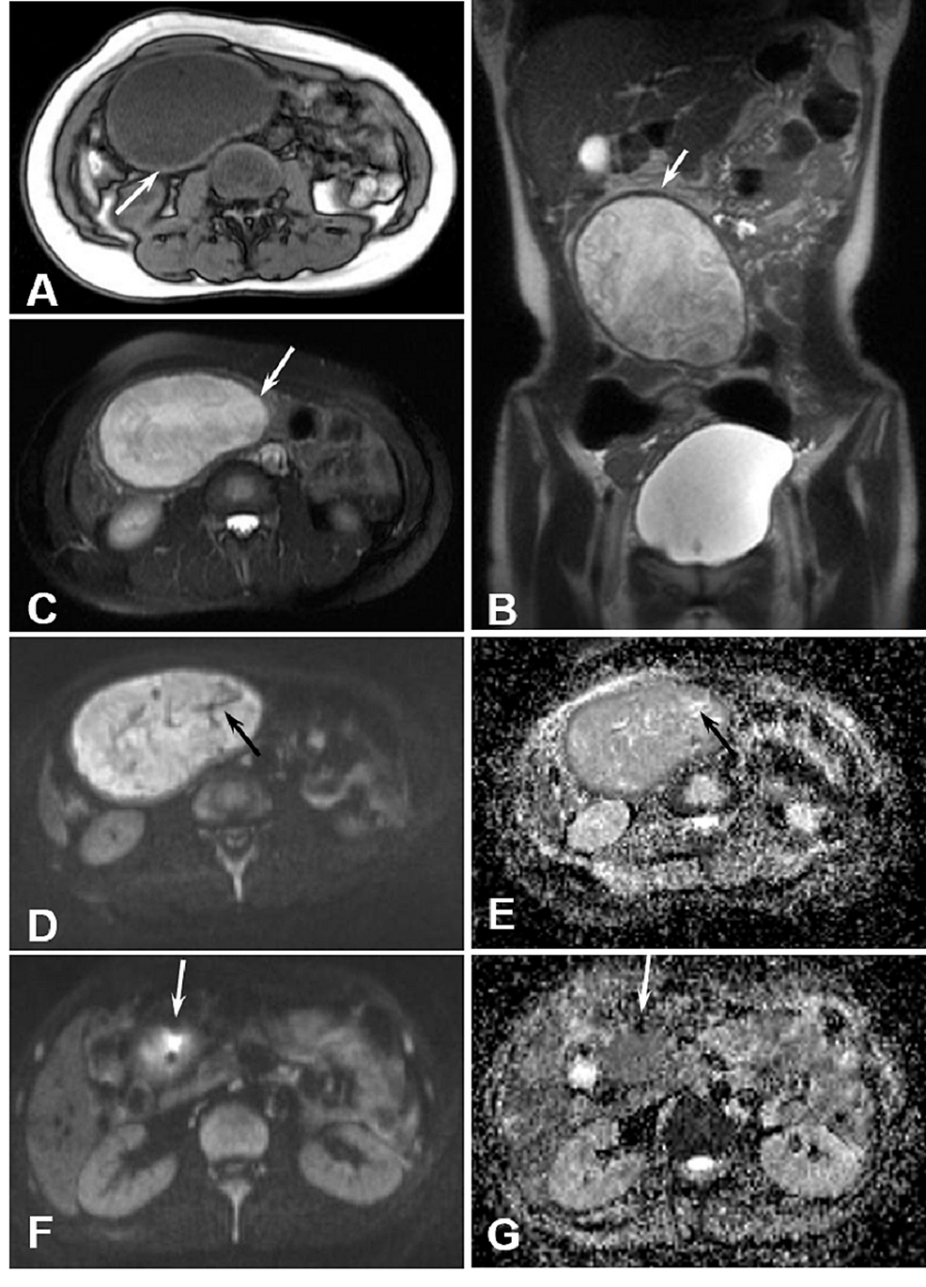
A 30-year-old woman with a mass in her abdomen revealed at five years after caesarean section. (A) Axial T1WI showed a round hypointensity mass in the right middle and lower abdominal cavity (white arrow). (B) Coronal T2WI and (C) axial T2WI-FS showed heterogenous hyperintensity with a complete hypointensity capsule (white arrows in B and C). (D) Axial DWI showed swirling and hypointensity strip band (black arrow) in the hyperintensity background. (E) Axial ADC showed slight hypointensity and strips of hyperintensity (black arrow) were seen inside. (F) Axial DWI showed the magnetic sensitive artifacts (white arrow). (G) Axial ADC corresponds to F.
